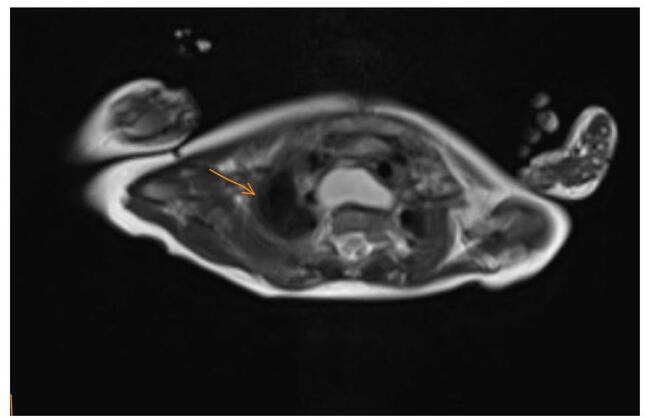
Axial chest computed tomography (CT) image demonstrating a well-defined, rounded mass in the mediastinum adjacent to the esophagus (orange arrow). The lesion exhibits homogeneous density and smooth margins. No significant compression of surrounding structures is observed. The findings were later confirmed to represent an esophageal duplication cyst upon further diagnostic evaluation.
